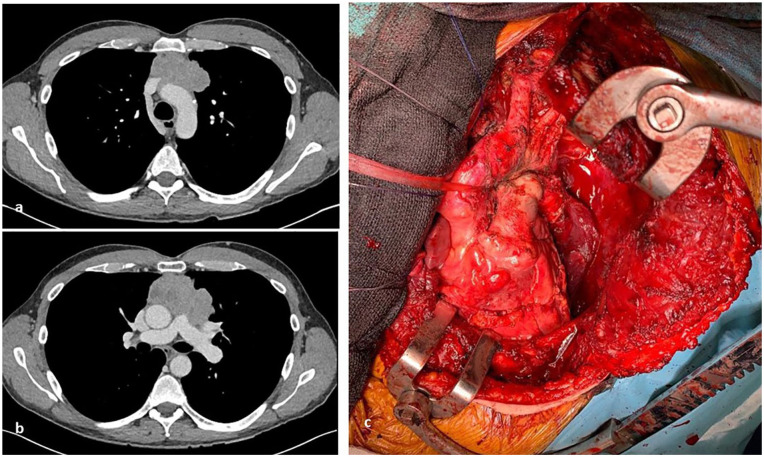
Preoperative computed tomography shows an anterior mediastinal mass with possible extension into the anterior aspect of great vessels (aortic arch, superior vena cava, left brachiocephalic vein, main pulmonary artery, proximal left pulmonary artery, left superior pulmonary vein) ([a] and [b]). Operative picture (c) demonstrates the great vessels following resection.
